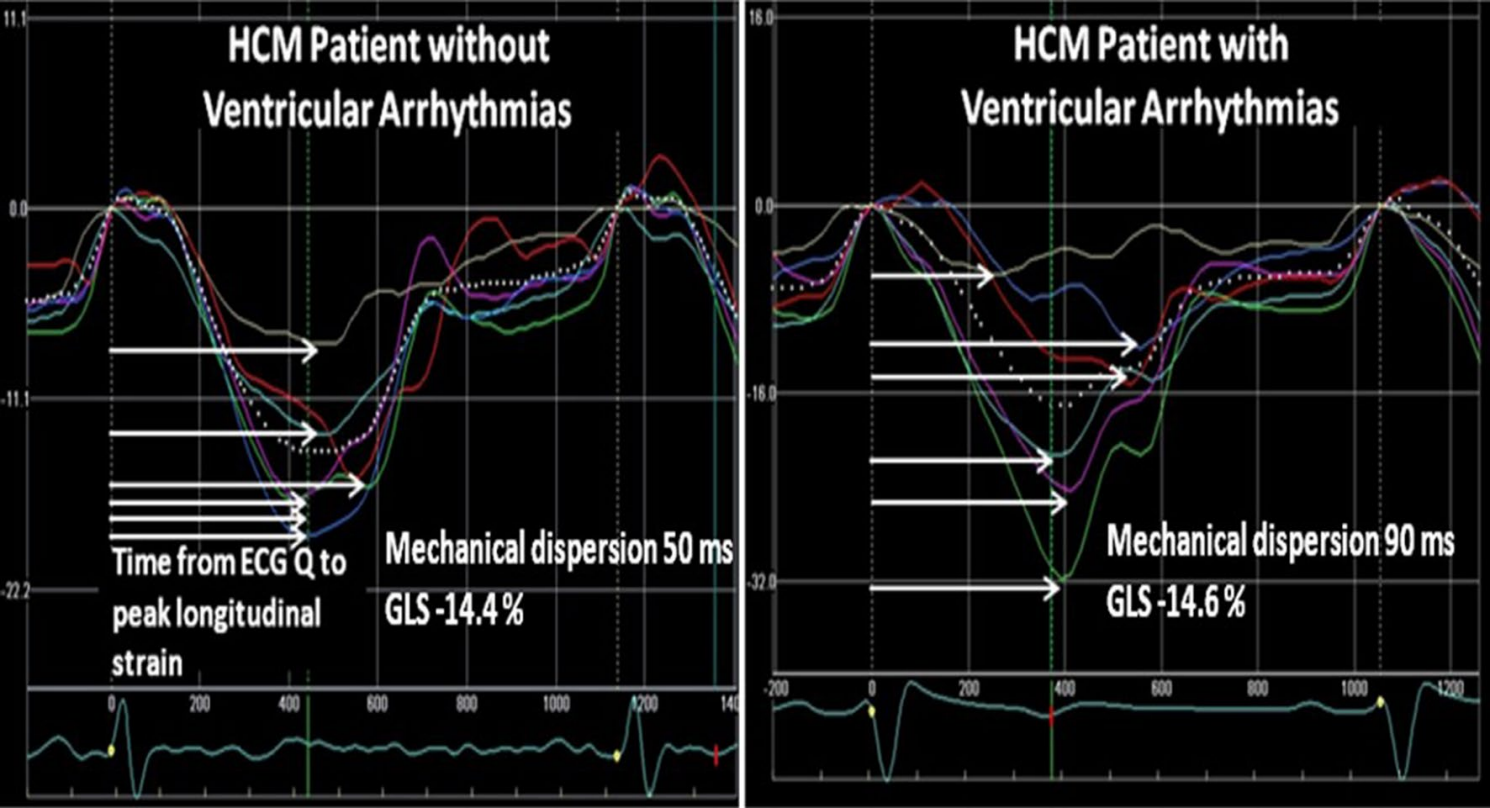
Mechanical dispersion by strain echocardiography in two patients with HCM. Horizontal white arrows indicate time to peak strain defined as the time from onset of Q/R to peak negative strain in each segment. Left panel displays longitudinal strain curves and mechanical dispersion in an HCM patient without ventricular arrhythmias. Left panel shows more pronounced mechanical dispersion in an HCM patient with ventricular arrhythmias
